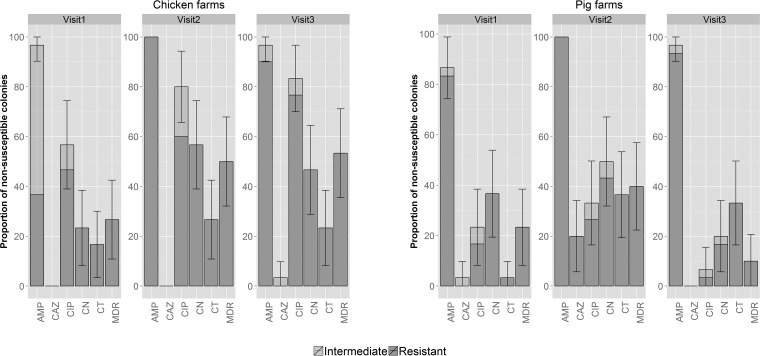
Prevalence of AMR among 180 E. coli isolates (30 isolates/visit/host species) from 12 farms in Tien Giang, Vietnam (2013–2014). The error bars indicate 95% confidence intervals. AMP, ampicillin; CAZ, ceftazidime; CIP, ciprofloxacin; CN, gentamicin; CT, colistin.
